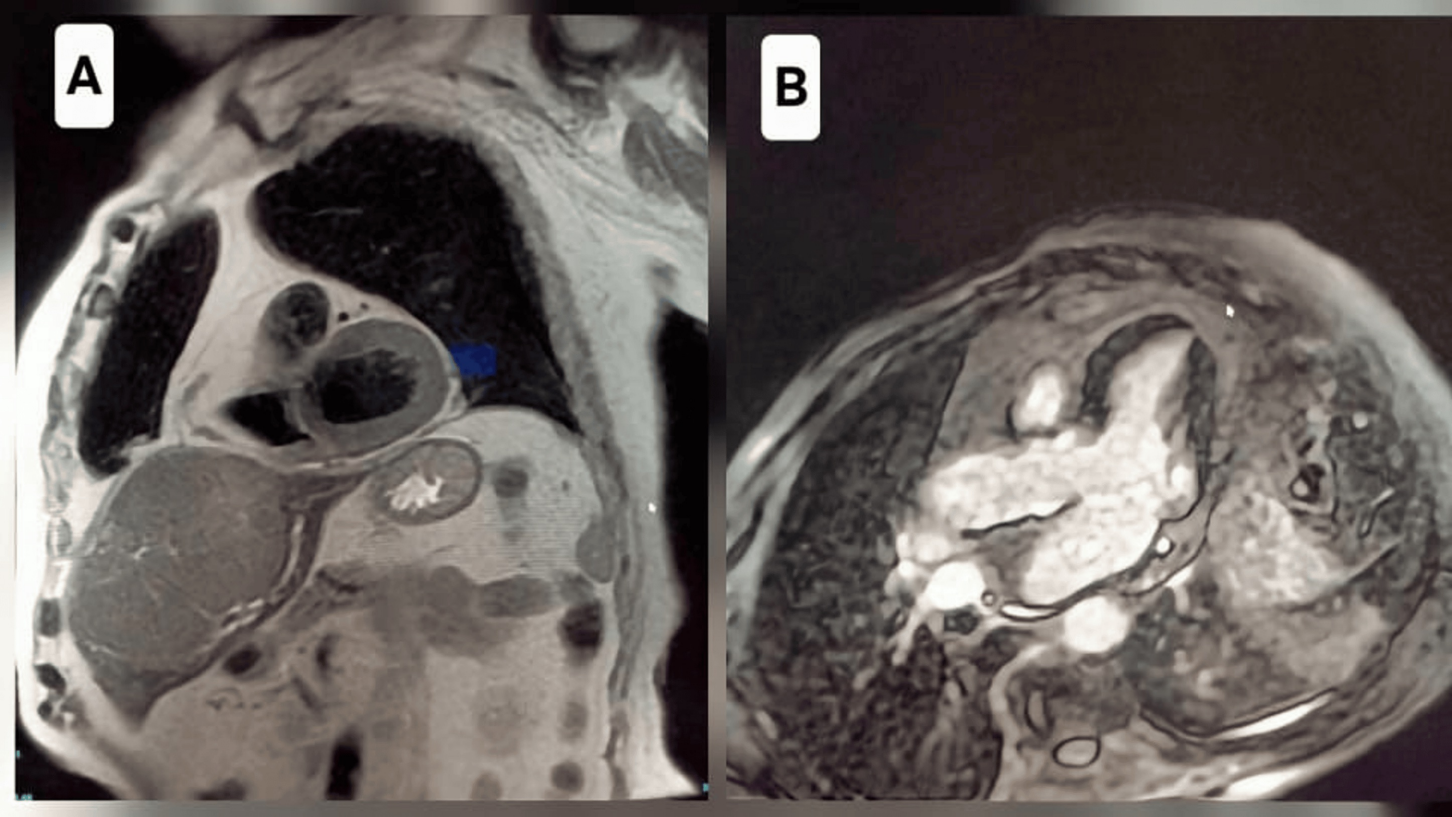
Cardiac MRI (A) T2 black-blood sequence, short axis view: homogeneous myocardial signal, without focal or diffuse hypersignal suggestive of myocardial edema. No inflammatory wall thickening or segmental signal abnormality; (B) Late gadolinium enhancement (LGE) sequence, three-chamber view: no focal or diffuse late myocardial enhancement, particularly in the subepicardial or intramural areas. There was no evidence of myocardial necrosis or inflammatory fibrosis.
